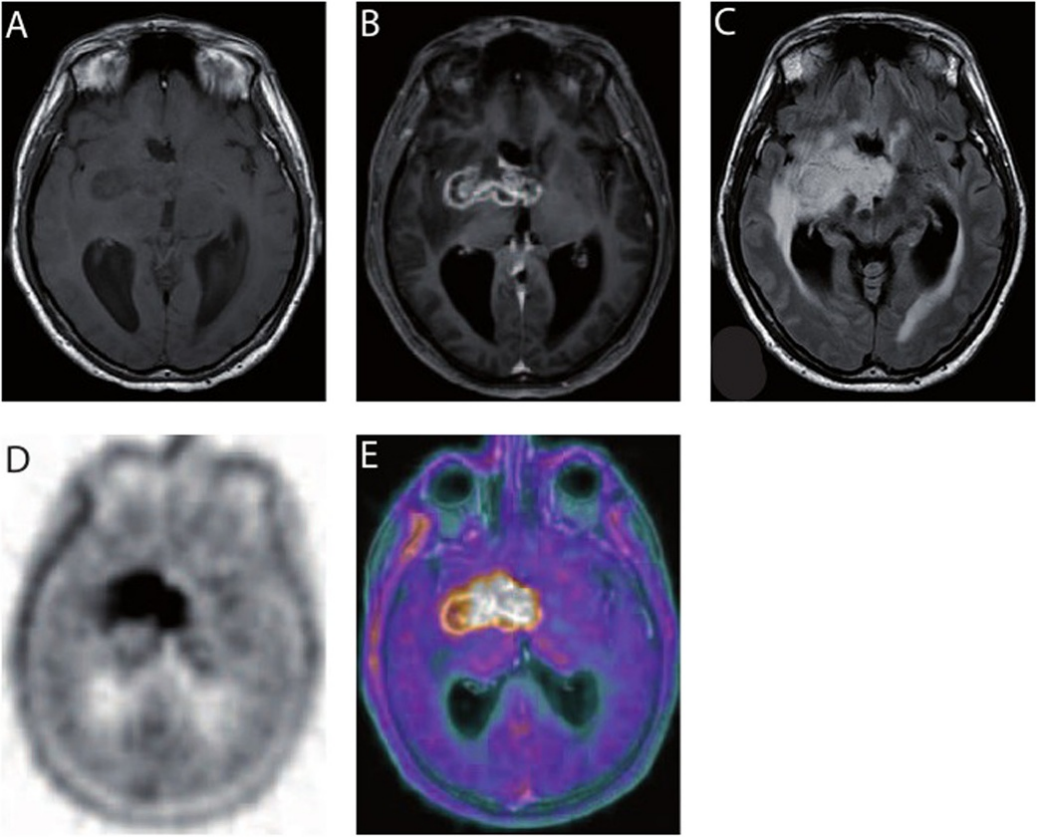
Relapse of GBM after resection and radiochemotherapy. A Native T1-weighted MRI shows a tumor involving the right basal ganglia. B Gadolinium-enhanced T1-weighted MRI shows strong peripheral and irregular enhancement of the tumor. C T2-weighted FLAIR demonstrates pronounced surrounding edema and mass effect. D In the PET/CT scan and E the fusion of PET and MRI, the lesion described in the MRI corresponds with focal increased ^18^ F-FET uptake exhibiting Tu/Bg ratio of 2.4.
